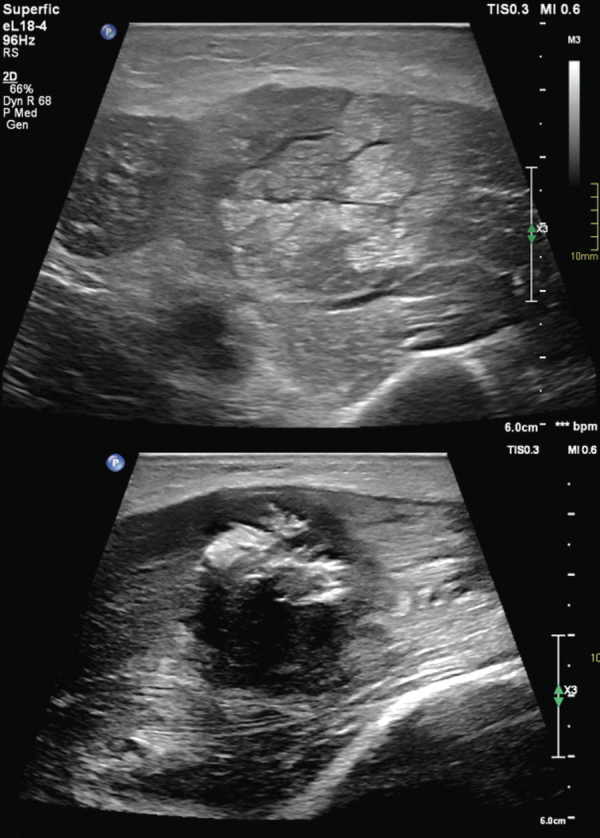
Ultrasound of myositis ossificans shows muscle oedema and calcifications.
